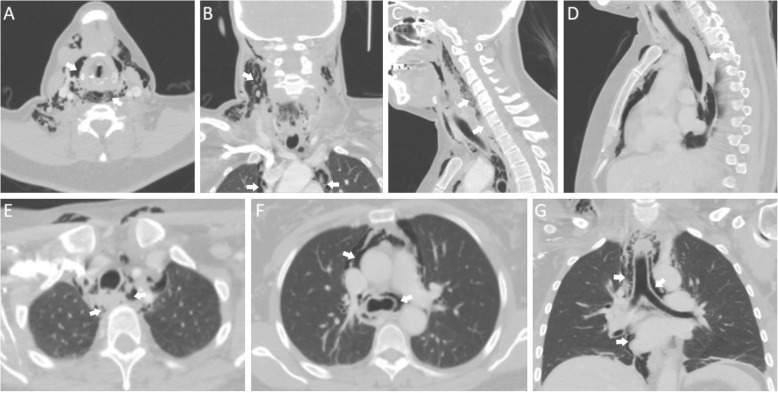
CT scan findings: a–b. Extensive emphysema in the soft tissues of the neck. Free air is also visible in the upper mediastinum (white arrows). c–d. Sagittal sections showing communication between the esophagus and a false lumen corresponding to the cervical and upper thoracic prevertebral space, containing air and small amounts of fluid (white arrows). e–g. Free air in the upper and middle mediastinum, along the intrathoracic esophagus and around the big mediastinal vessels (white arrows)
